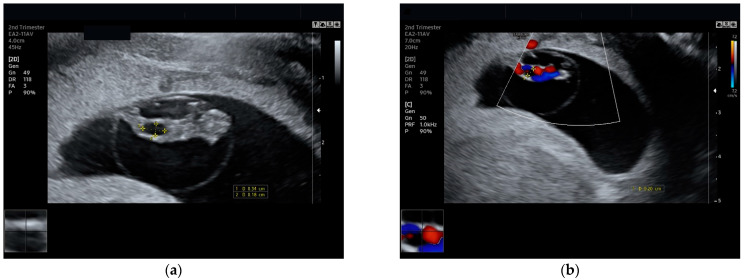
Umbilical cord cyst: (a) 0.34/0.18 cm, diagnosed in the first trimester; (b) Doppler image showing a small transsonic area, consistent with a small umbilical cyst.
